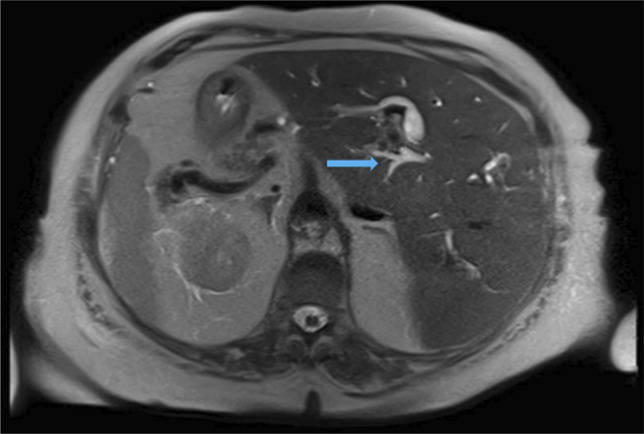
Axial magnetic resonance imaging demonstrates intrahepatic biliary tree dilatation (arrow). Transposition of the liver, spleen, and pancreas are noted.
